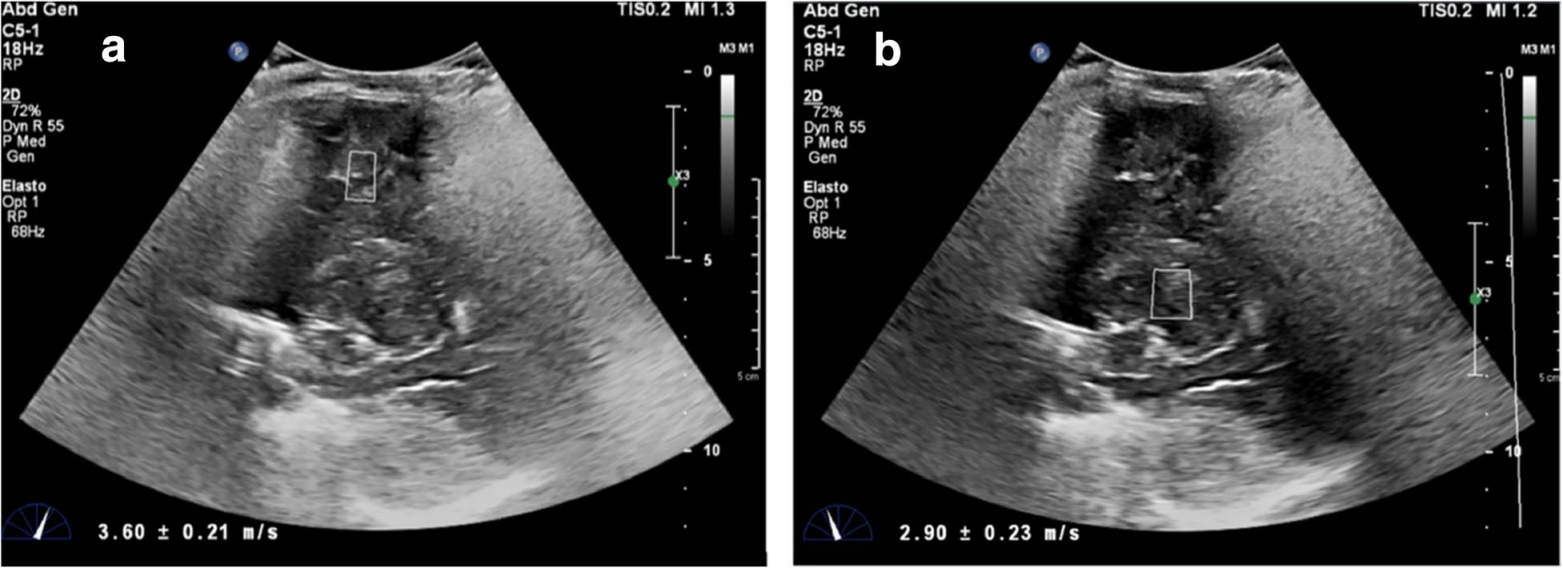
Ultrasound elastography of an infant with severe anoxia. This is a 6-month-old, former 37-week gestational infant with unwitnessed cardiac arrest at home. Capillary gas pH 6.8, pCO2 43, pO2 144, and bicarbonate 3.5. End tidal CO_2_ during resuscitation were in the 20 s and high 40 s. He was imaged 30 min after return of spontaneous circulation. Values were elevated in the gray and white matter 3.6 ± 0.21 m/s (a) and basal ganglia 2.9 ± 0.23 m/s (b). (Reproduced from deCampo and Hwang [130] with permission.)
